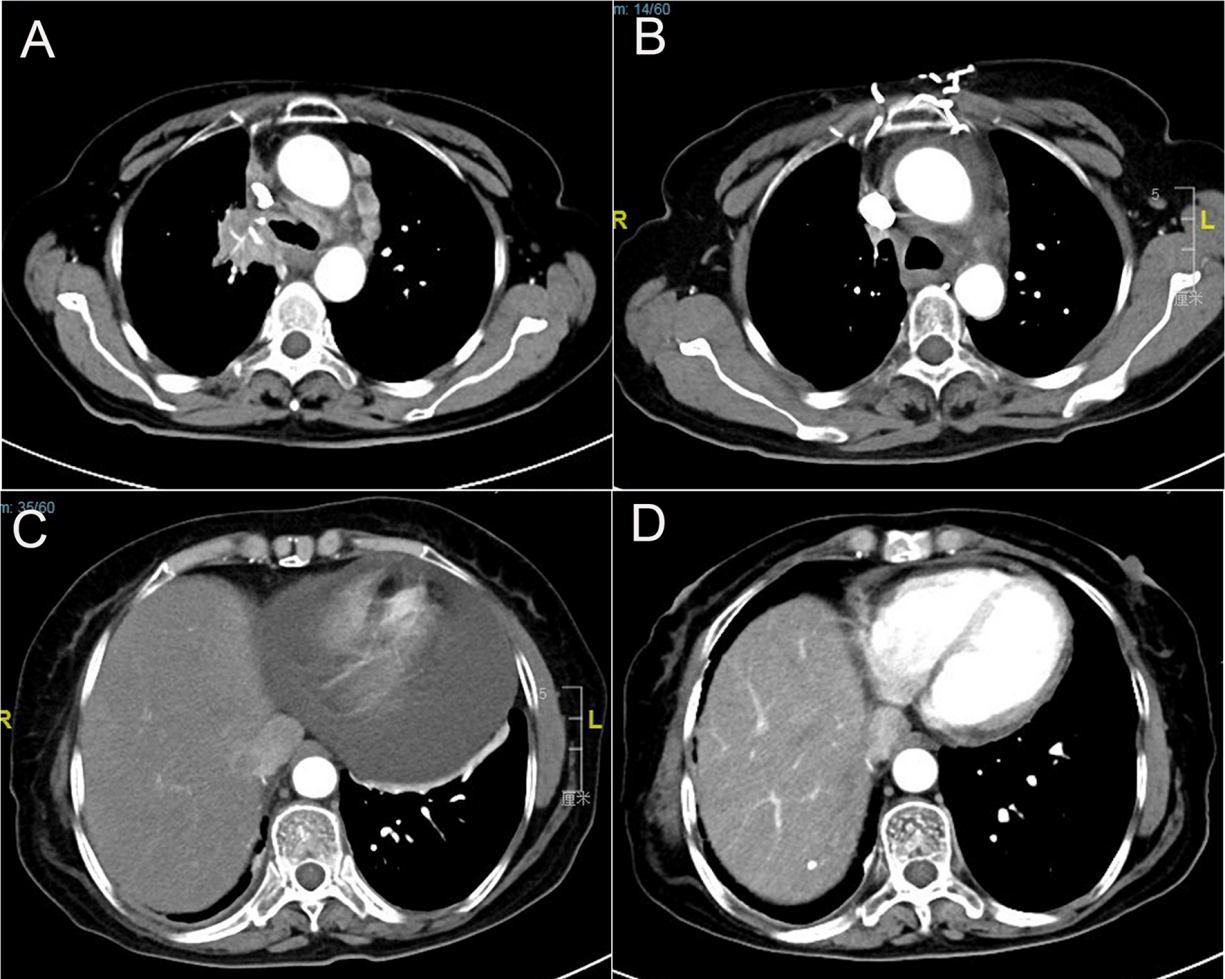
Computed tomography scans show (A) lung lesions and mediastinal lymph node metastasis before icotinib therapy; (B) partial response in lung lesions after icotinib treatment; (C) pericardial effusion development; and (D) pericardial effusion was well controlled after osimertinib treatment.
